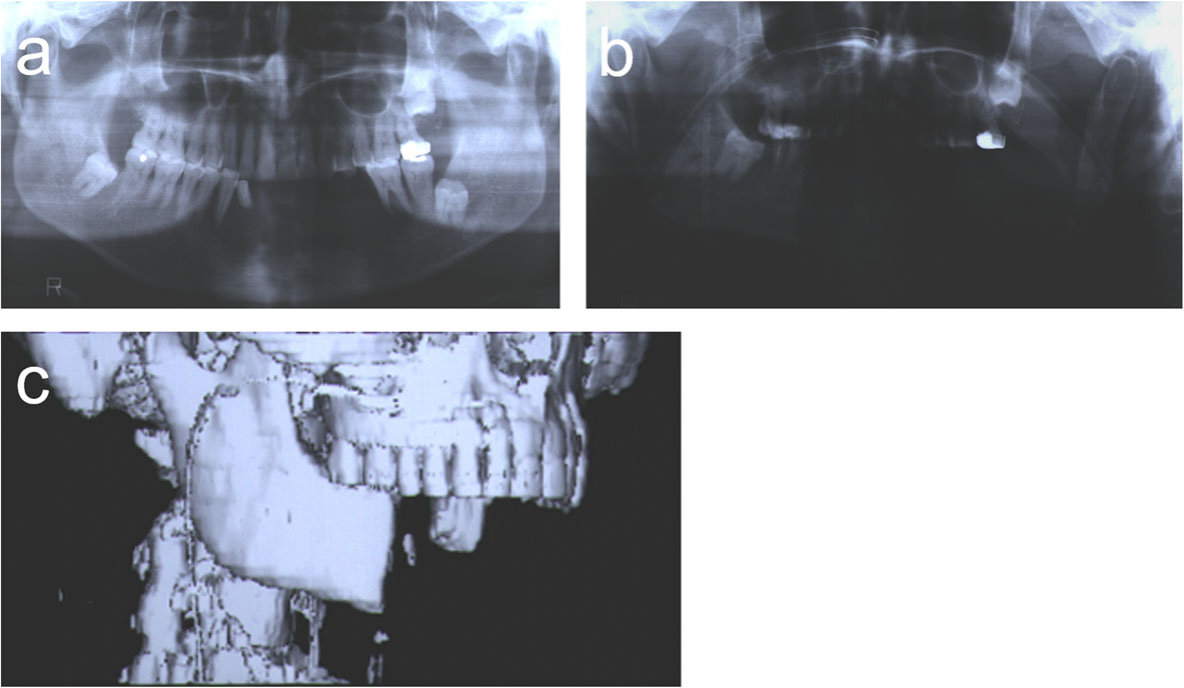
a Orthopantomogram before treatment. b Orthopantomogram and c 3-D computed tomography scan after segmental mandibular resection
